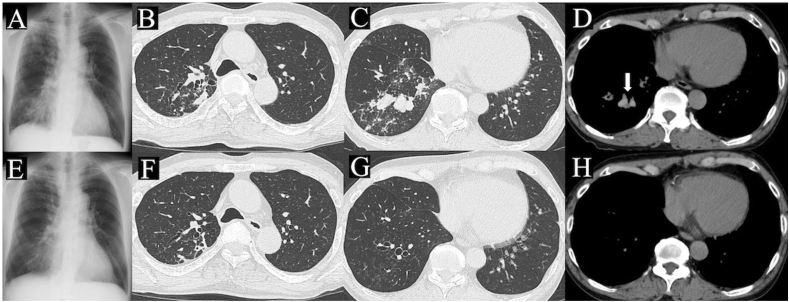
Chest radiograph of the patient, acquired at the initial visit to our hospital, showing consolidations in the right upper and lower pulmonary fields (A). Chest CT scan of the patient showing worsening consolidations in the upper lobe of the right lung (B), central bronchiectasis, and centrilobular nodules in the lower lobe of the right lung (C). HAM suggests allergic bronchopulmonary aspergillosis evident in the lower lobe of the right lung (D) (arrow). Chest radiograph acquired one month after treatment initiation shows disappearance of consolidations in the right upper and lower pulmonary fields (E). Chest CT image showing a reduction in consolidations and nodules in the upper (F) and lower lobes of the right lung (G). HAM in the lower lobe of the right lung disappeared (H). CT: computed tomography; HAM: high attenuation mucus.
